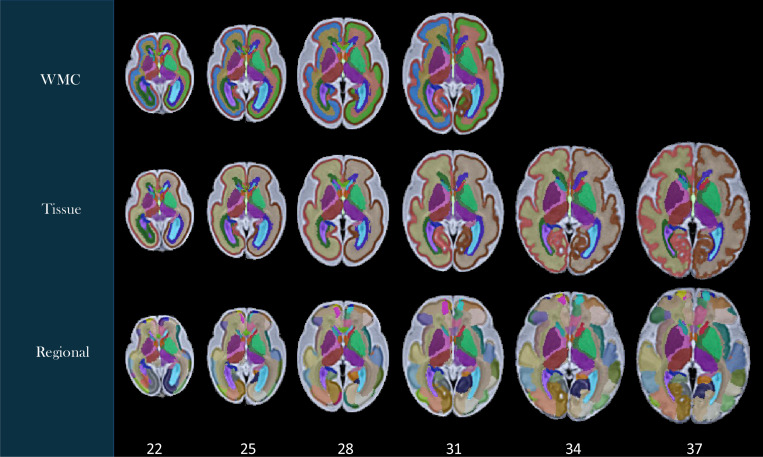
Tissue and regional segmentations and structural labels overlaid on axial views of the CRL-2025 spatiotemporal fetal brain MRI atlas at six representative gestational age (GA) weeks. All label schemes have subcortical structures including lentiform and caudate nuclei, internal capsules, thalami, and hippocampi separately on each hemisphere. Tissue segmentation labels (middle row) delineate the cortical plate-white matter boundary, CSF, and subcortical structures. In the top row, the white matter of the tissue segmentation is divided into white matter compartments (WMC) including the ventricular and intermediate zones and the subplate. These transient WMCs gradually disappear and were not clearly observable on the atlases beyond 31 weeks. The bottom row displays regional segmentations which are useful for regional and connectivity analyses.
